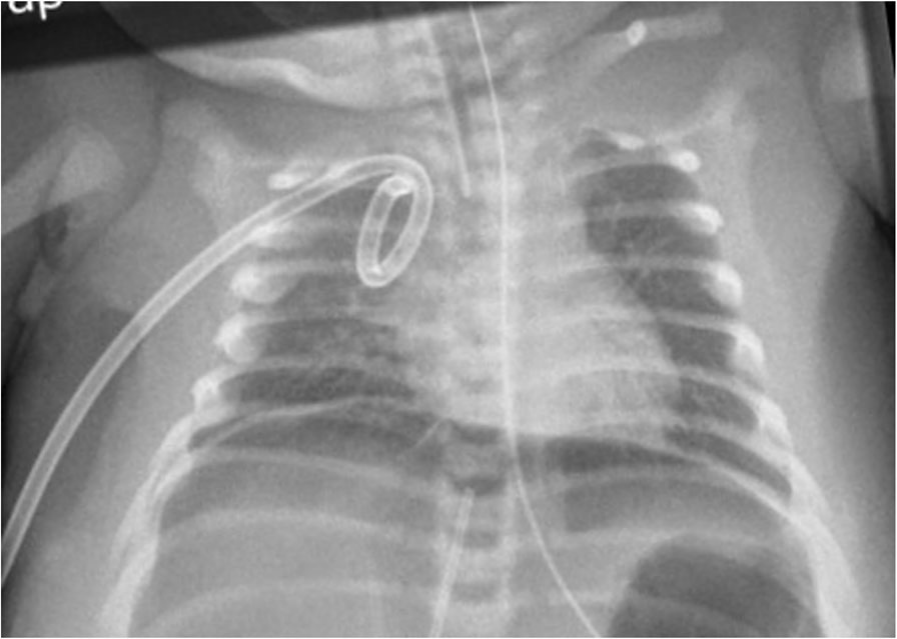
Chest X-ray depicting free abdominal air due to gastric perforation
